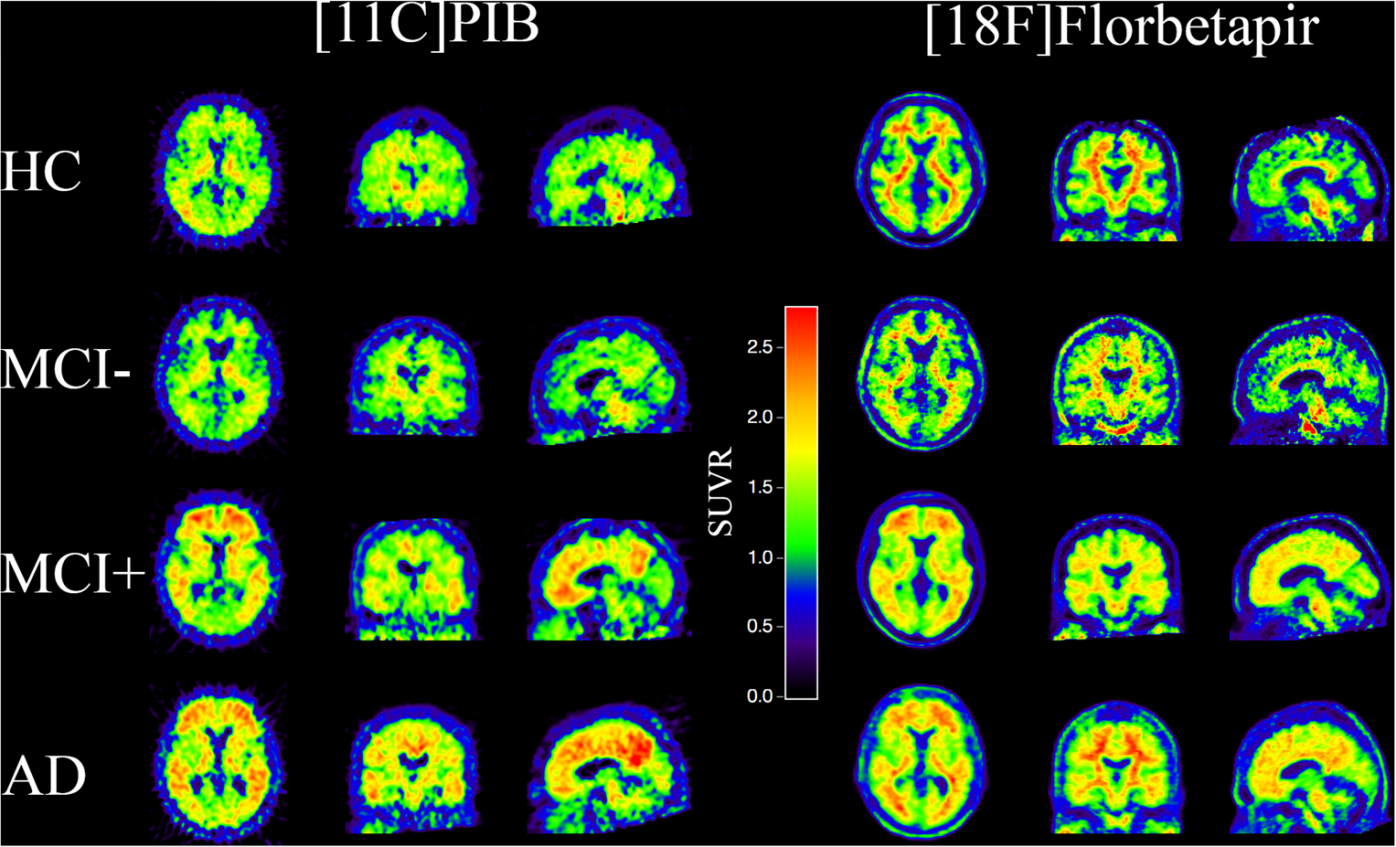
Typical examples of individual SUVR images from HC, and MCI amyloid-negative (MCI-), MCI amyloid-positive (MCI+) and AD patients matched for age, gender and MMSE. [11C]PIB scans are on the left and [18F]Florbetapir scans are on the right. Amyloid positivity has been defined as a CCTXR value above the cut-off points of 1.42 for [11C]PIB and 1.34 for [18F]Florbetapir
